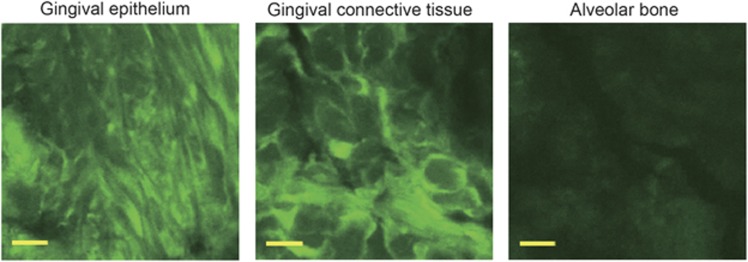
Magnified views of periodontal tissue in the high-intensity ultrasound-microbubble (HUM-Sc) group. High magnification of the gingival epithelium (E), gingival connective tissue (C), and alveolar bone (B) in Figure 2. Scale bar=10 μm.
